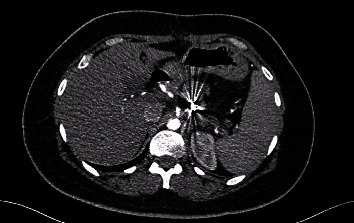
Post-embolization control CT scan at 3 months, metal coils placed. CT, computer tomography.
